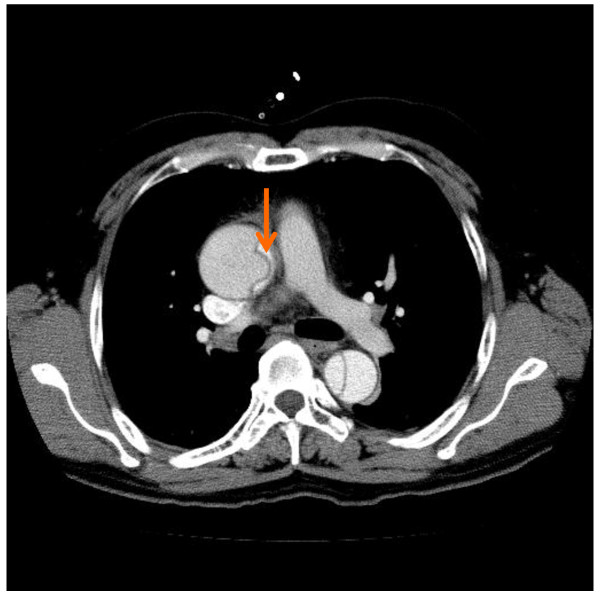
Typical preoperative computed tomography. The arrow indicates the estimated direction of needle insertion into the true lumen.
